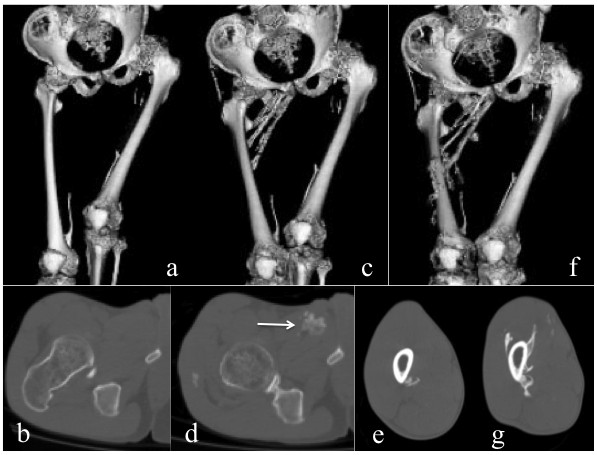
CT images from Case 2. Reconstructed 3D-CT images of the pelvis and bilateral femora at baseline (a), at M-12 m (c), and at D-12 m (f). Axial CT images of the right hip at baseline (b), and at M-12 m (d), and those of the right mid-femur at M-12 m (e), and at D-12 m (g). Heterotopic ossifications in the right adductor muscle (arrow) developed during the medication phase and around mid-femur developed during the discontinuation phase ware clearly demonstrated.
